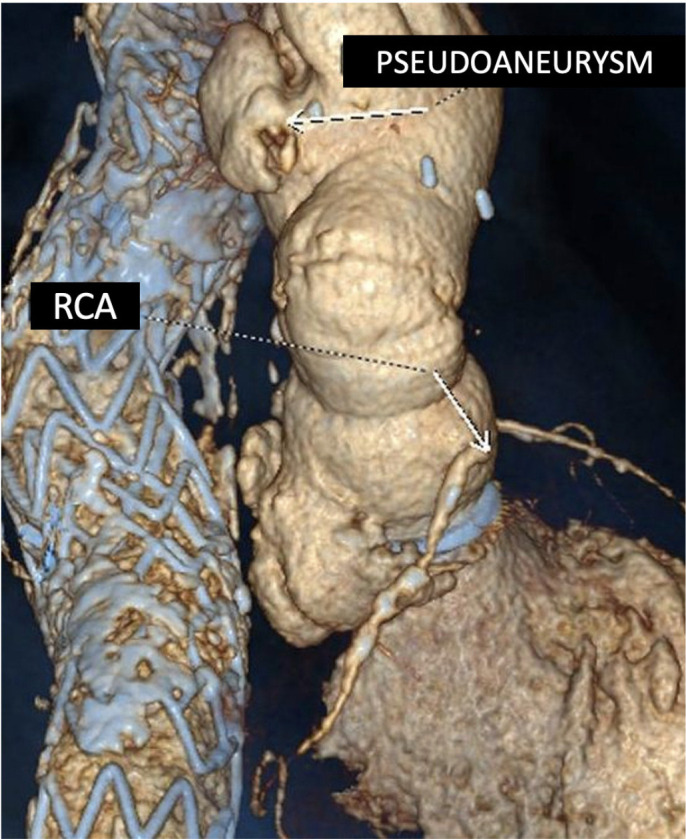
Computed tomography scan showing three aortic pseudoaneurysms: a proximal
